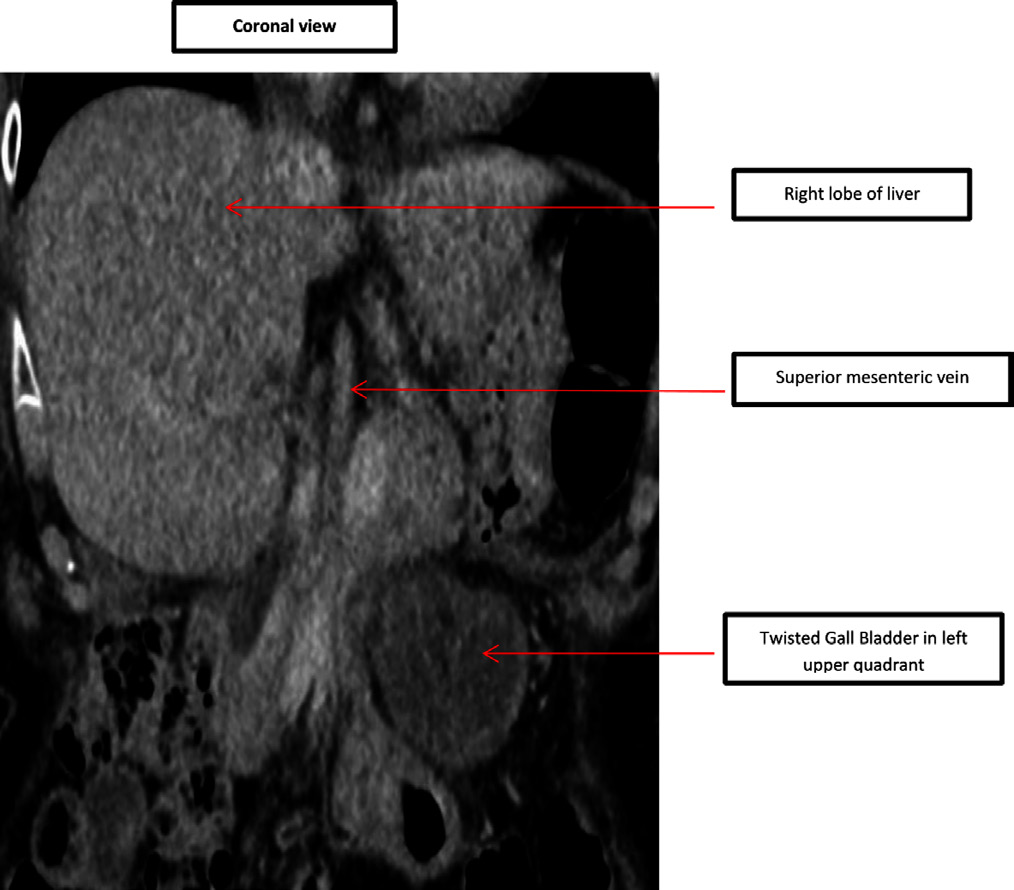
CT scan - Coronal view demonstrating twisted gall bladder in left upper quadrant
